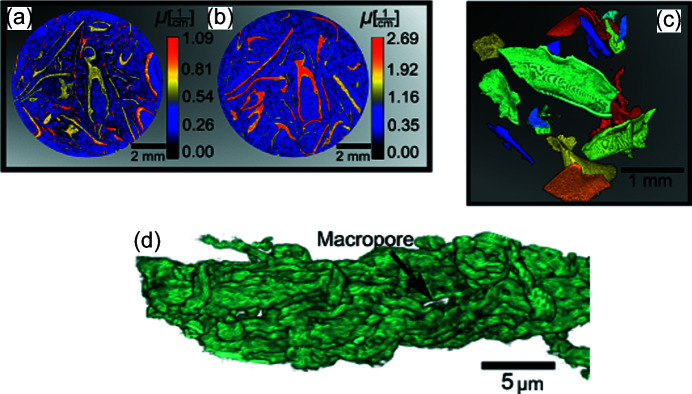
(*a*) X-ray tomogram of SBA-15 1:1 showing clusters with different morphologies than observed for smaller ratios of HBsAg to SBA-15, obtained using the X-ray mCT setup. (*b*) Neutron tomogram of the SBA-15 1:1 sample showing clusters assigned to HBsAg with the same shape observed with X-rays, obtained with the CONRAD instrument. (*c*) 3D visualization of HBsAg aggregates in the SBA-15 1:1 sample. Each aggregate is shown with a different colour. These aggregates have a plate shape which is not observed in any other SBA-15 to HBsAg ratios. (*d*) 3D visualization of the SBA-15 particle using ID16B setup. The silica rods and some larger macropores can be observed, as indicated by the arrow (white region).
